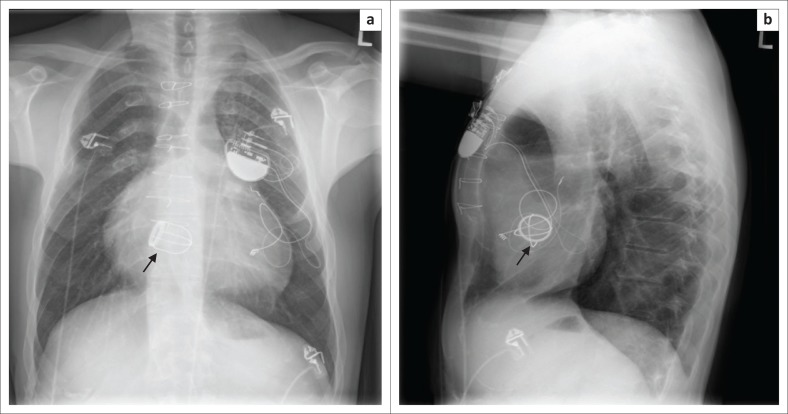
Frontal (a) and lateral (b) chest radiographs showing an isolated prosthetic tricuspid valve replacement (Starr Edwards valve) (arrow) in a 51-year-old male patient with a history of Ebstein’s anomaly. Note the implanted pacemaker with its epicardial leads.
